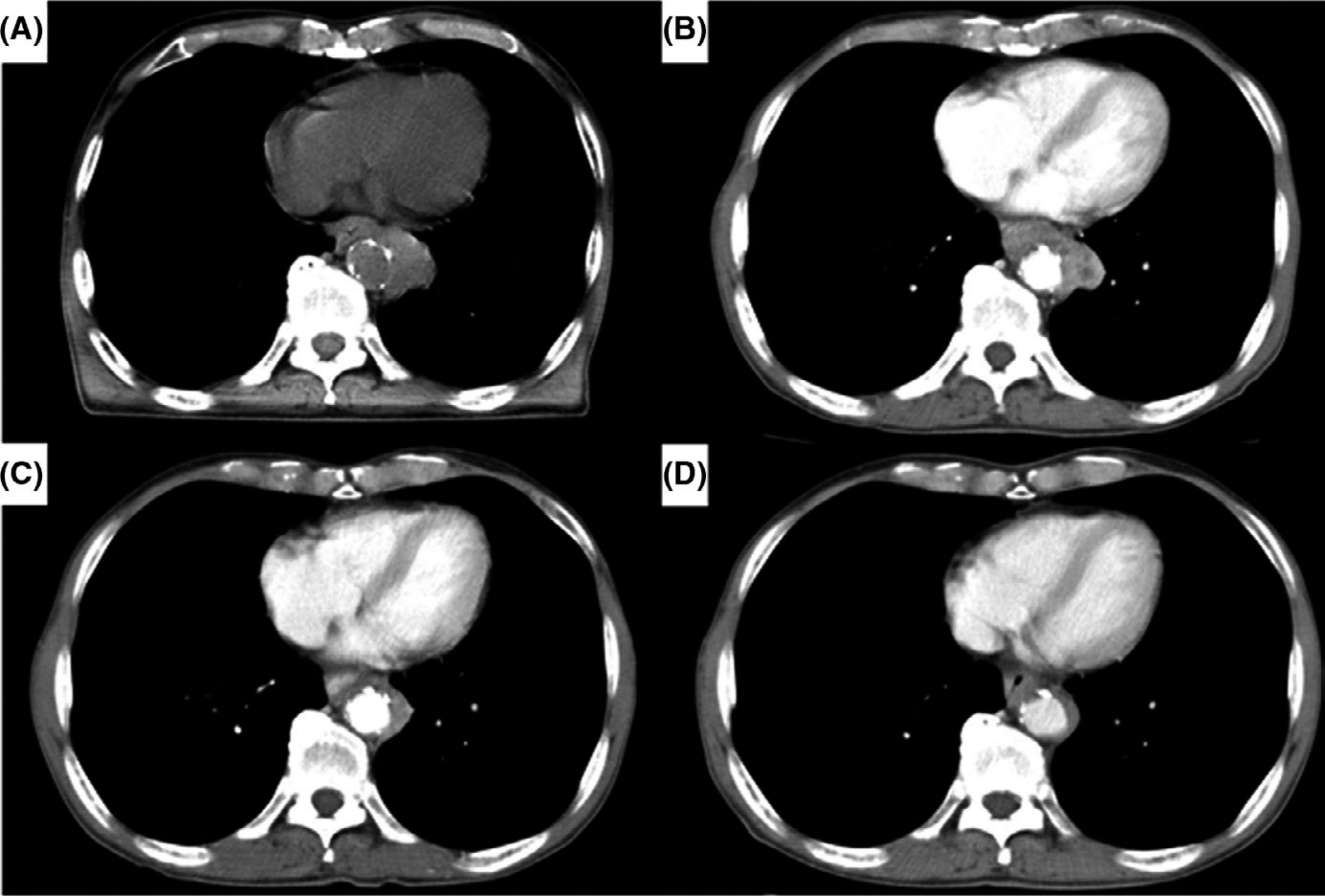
Patient who underwent R0 curative resection after induction therapy (5‐fluorouracil+cisplatin:FP + 40 Gy). A, Primary tumor and metastatic lymph node invading the aorta before induction therapy in non‐contrast‐enhanced computed tomography (CT) scan. B, Primary tumor and metastatic lymph node invading the aorta before induction therapy in contrast‐enhanced CT scan. C, The tumor immediately after induction therapy. D, The tumor after about 4 wks after induction therapy
